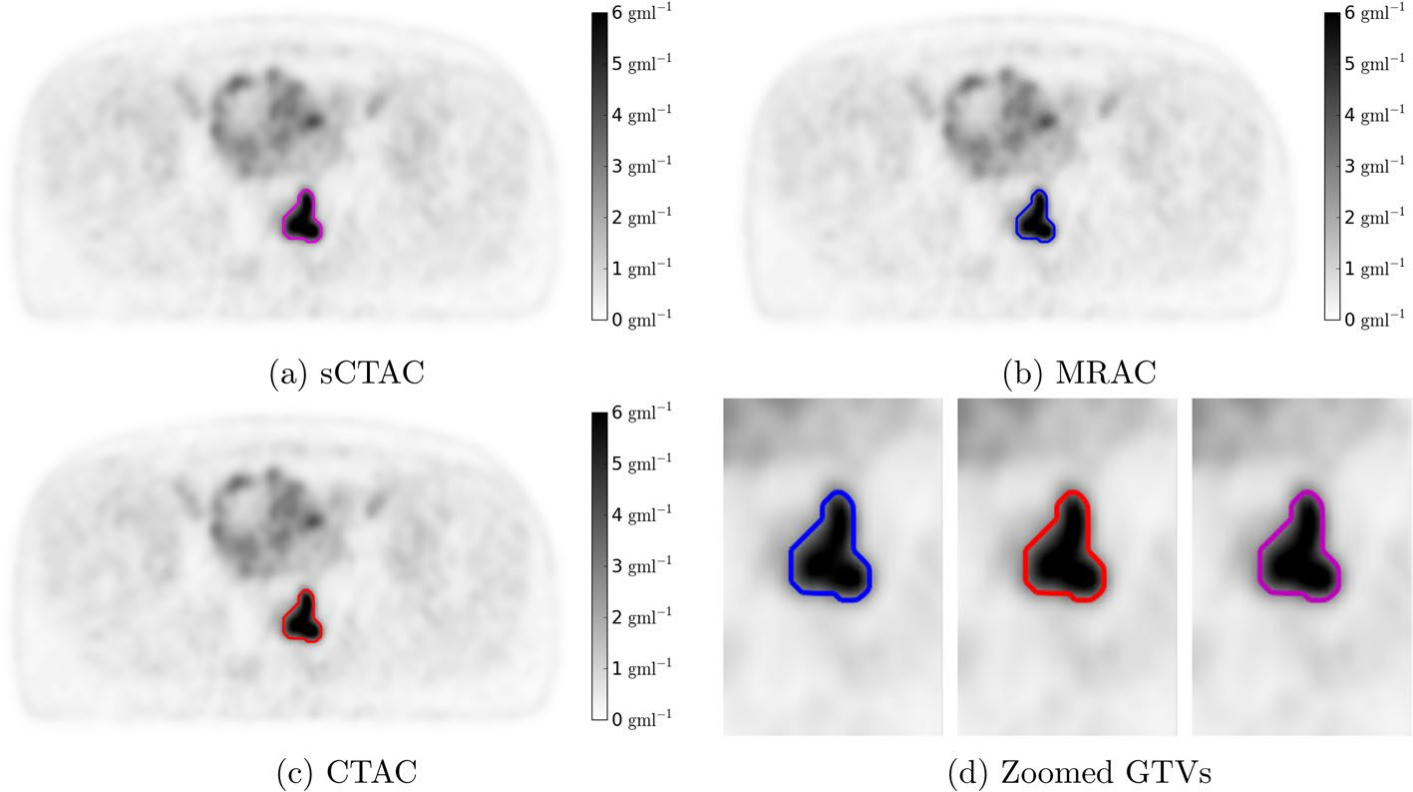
Example PET images reconstructed using the sCTAC (a), MRAC (b) and CTAC (c) attenuation correction maps. The threshold GTV contour is shown in purple, blue and red, respectively. Zoomed in pictures of the same GTVs are shown in (d). The patient was selected as having the sCTAC and MRAC $${\mathrm {SUV}}_{\max}$$ differences closest to the mean differences
